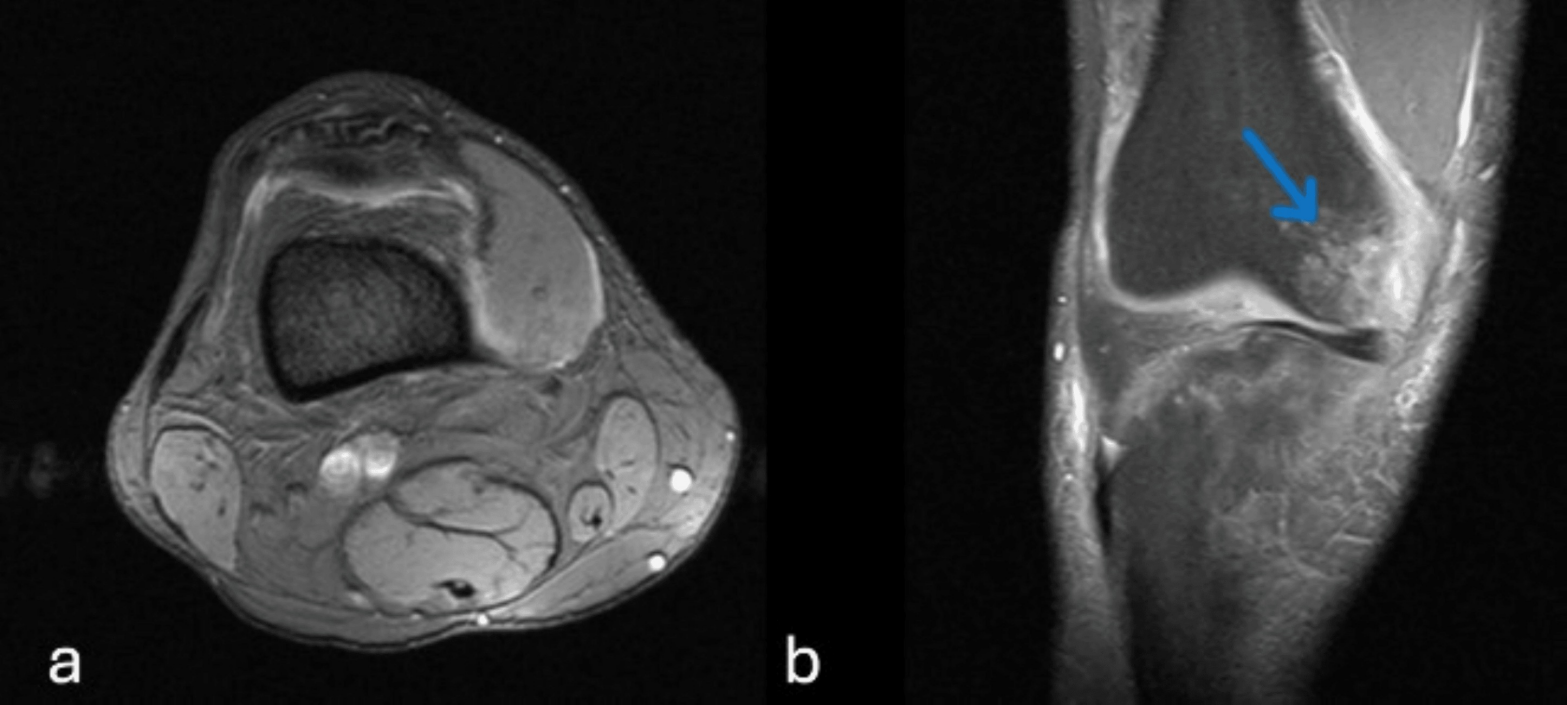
MRI of the right knee a) axial section shows no evidence of hemangioma in the vastus medialis muscle or intra-articularly; b) coronal section shows a bruise involving the medial femoral condyle (blue arrow points to the hyperintense signal)
